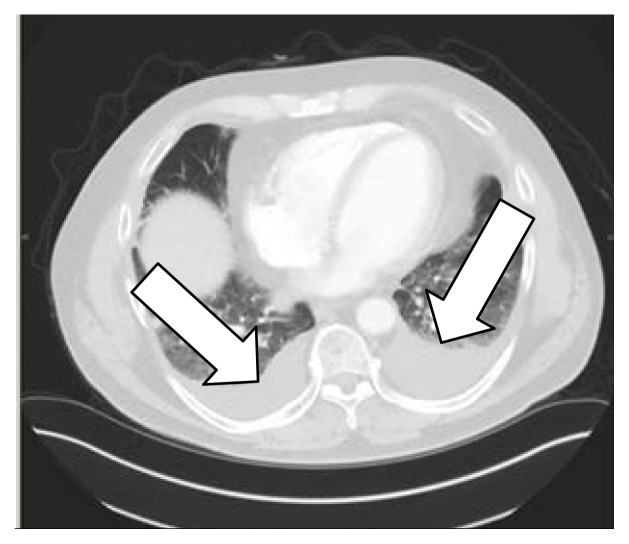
CT chest showed small to moderate right and moderate left pleural effusion
